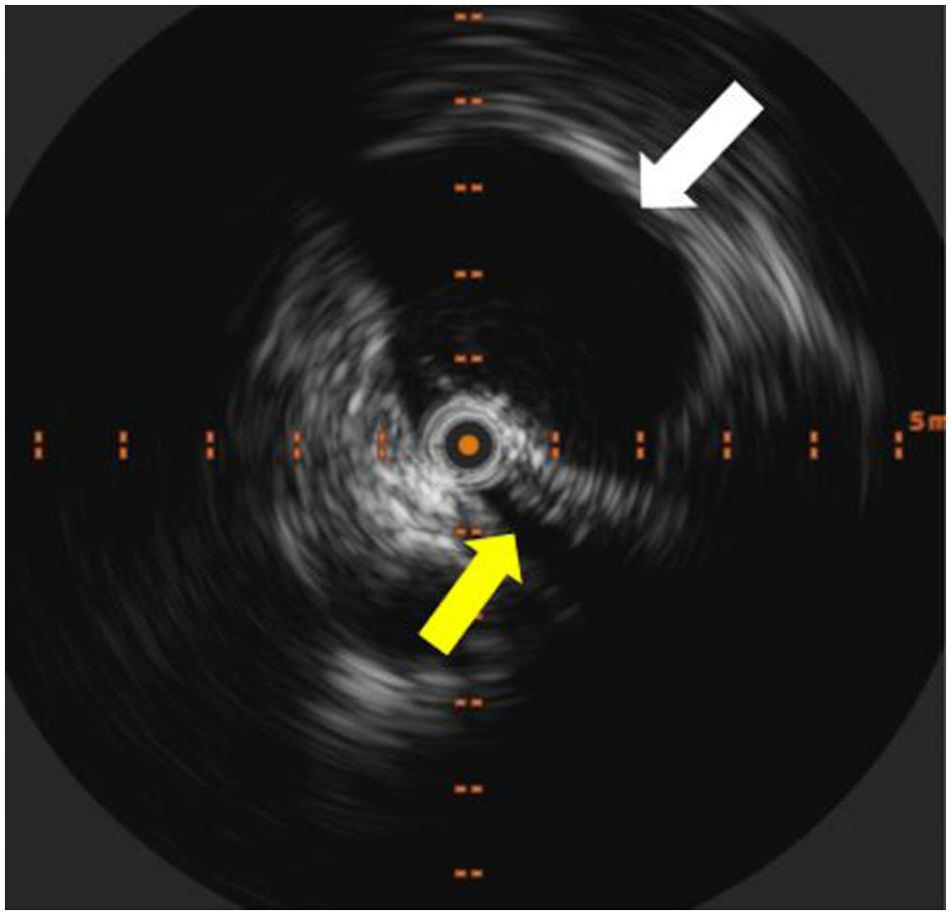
Intravascular ultrasound (IVUS) demonstrates severe LCIV compression (yellow arrow) by the overlying RCIA (white arrow). Abbreviation: LCIV = left common iliac vein; RCIA = right common iliac artery.
